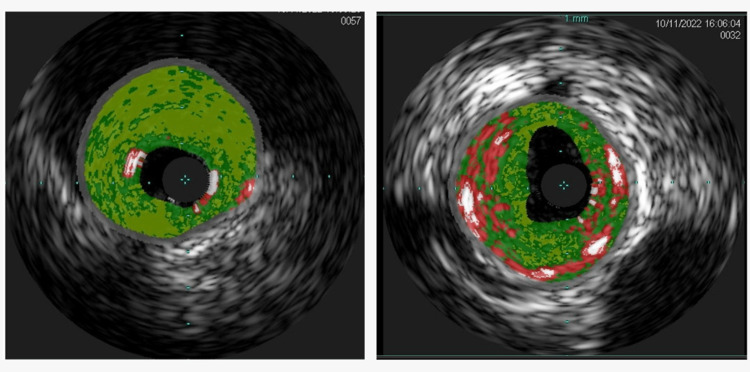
Intra-coronary (left anterior descending) ultrasound post-angiography revealing the underlying atherosclerotic disease.
